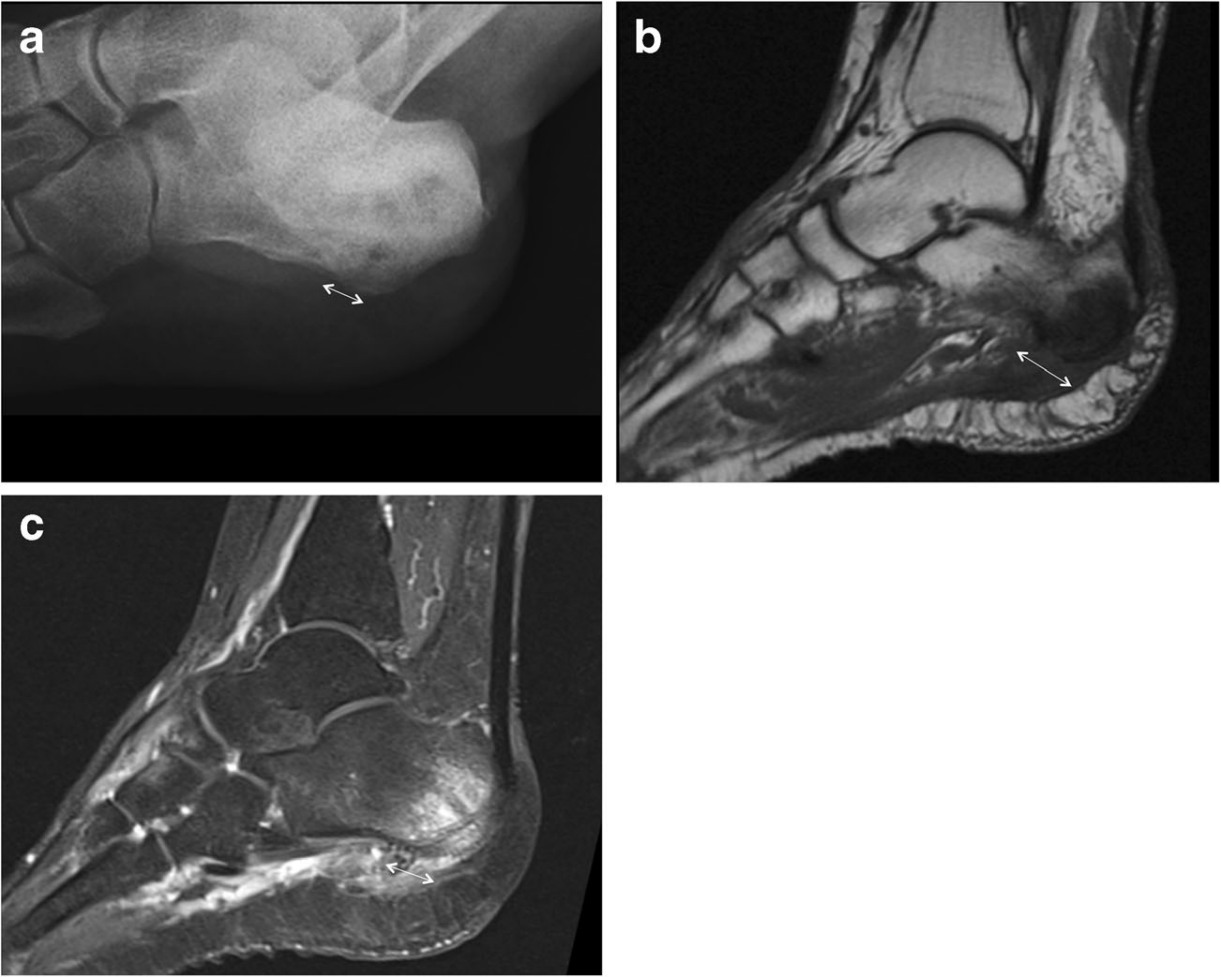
Heel osteomyelitis. Lateral plain radiograph shows marked morphological alteration of the heel with irregular lytic areas and concomitant PF thickening (*double-head arrow*) due to spreading of the infection (a). MRI confirms morphological alterations of the heel and PF (*double-head arrow*) on both T1-weighted (b) and fluid-sensitive (c) images
